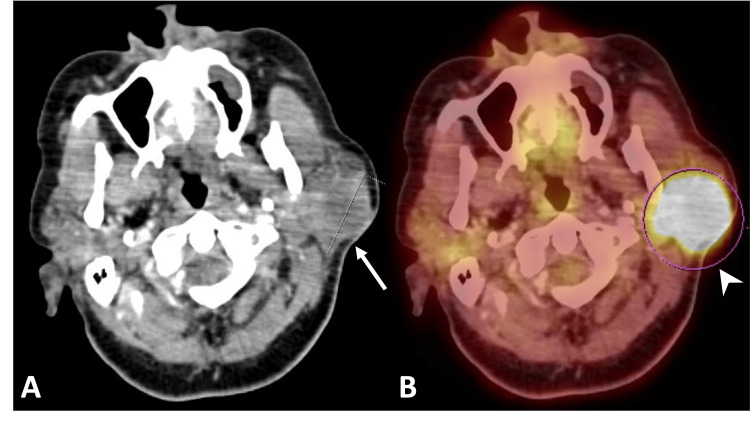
Breast cancer metastasis to the parotid gland. (A) Axial contrast-enhanced CT of the neck shows a large, lobulated, heterogeneously enhancing mass (white arrow) involving the superficial lobe of the left parotid gland. (B) 18F-FDG PET-CT demonstrates that the mass is markedly FDG-avid/hypermetabolic (arrowhead), with a maximum standardized uptake value (SUVmax) of 20. ^18^F-FDG PET-CT: Fluorine-18 fluorodeoxyglucose positron emission tomography/computed tomography; CT: Computed tomography; SUVmax: Maximum standardized uptake value
